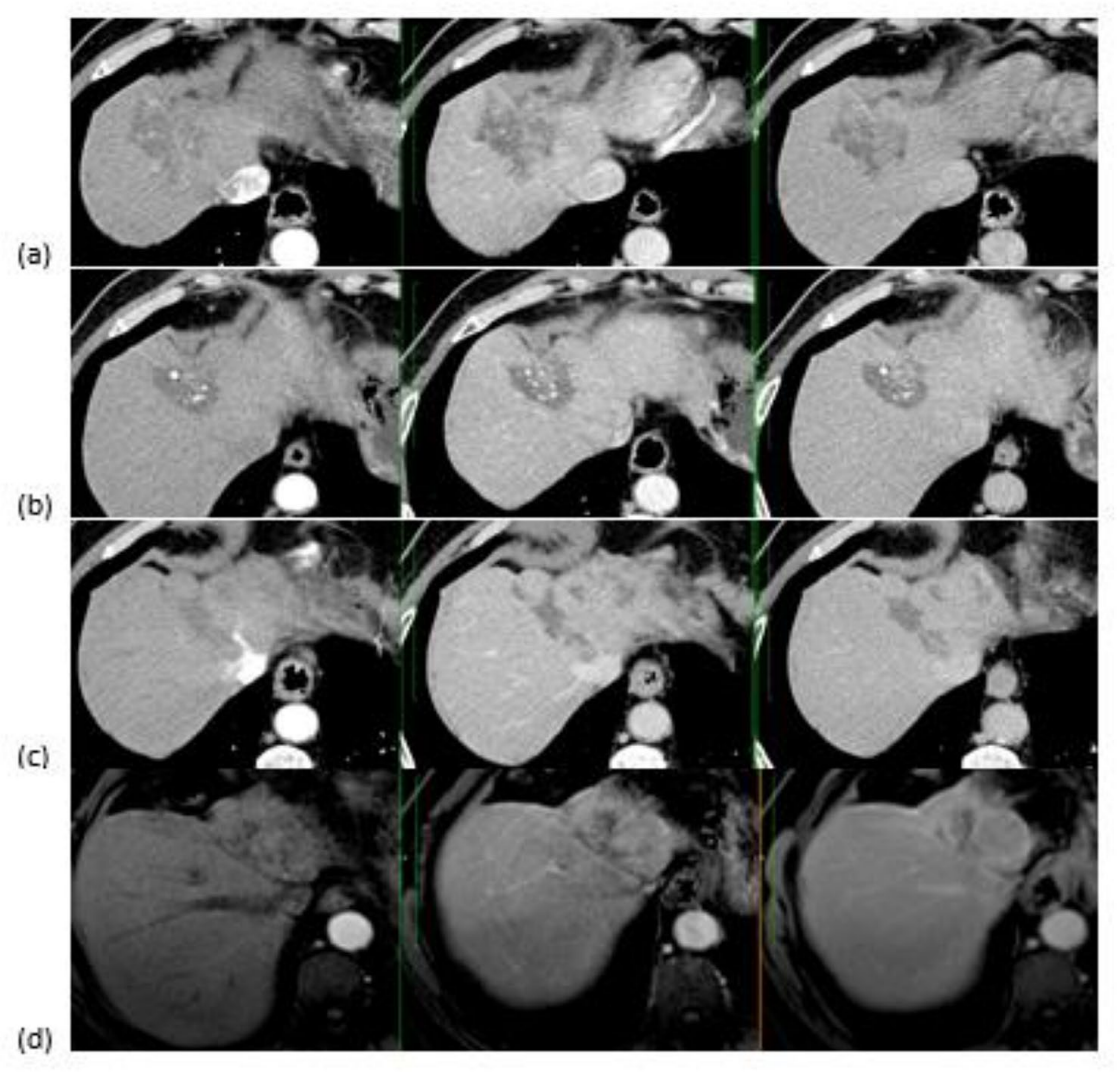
A case of male patient in the seventh decade of life with left lobe HCC (a) Triphasic CT before management demonstrating arterial enhancement & delayed washout within the lesion. (b) Follow-up triphasic CT after TACE revealed no residual activity within the managed lesion. (c) Follow-up triphasic CT 12 months after TACE revealed newly developed left lobe infiltrative HCC. (d) Patient received Sorafinib and follow-up dynamic MRI after 6 months revealed atrophy of the left lobe with complete tumor resolution & no residual or recurrent activity. In subsequent follow-ups on sorafenib, he continued to show a complete response
